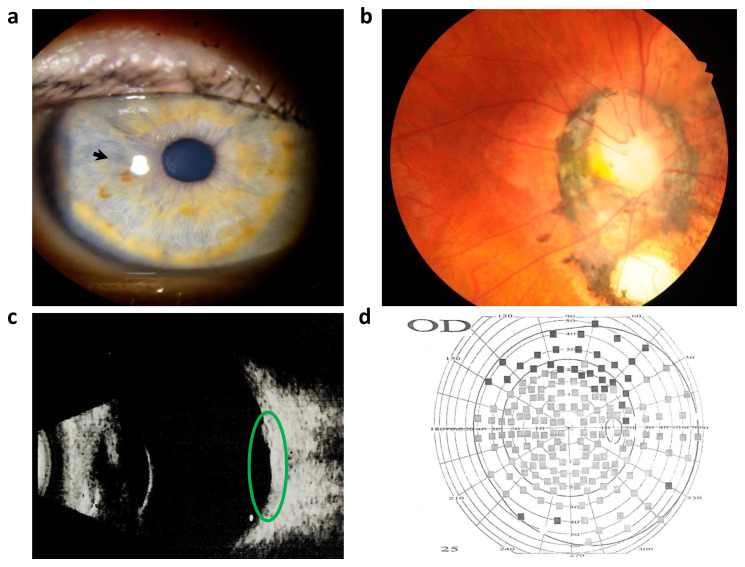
Ophthalmologic examination of the right eye. (a) Slit lamp examination depicted a radial elliptical anterior iris stromal defect (black arrow). (b) Retinal imaging conducted by a fundus camera displayed a pale pink, rounded optic disc with clear borders. An optic disc coloboma measuring half of the optic disc’s size was observed, accompanied by a notable deposit of black pigment. The macular zone exhibited an indistinct boundary. (c) Ultrasound B-scan of the right eye, focusing on the congenital optic disc coloboma (green ellipse), revealed clear optical media, membranes with adherent properties, and no presence of additional inclusions. (d) The result of computerized perimetry conducted on the right eye (OD) revealed narrowing of the peripheral borders of the visual field and relative scotoma up to 10 degrees from the fixation point.
